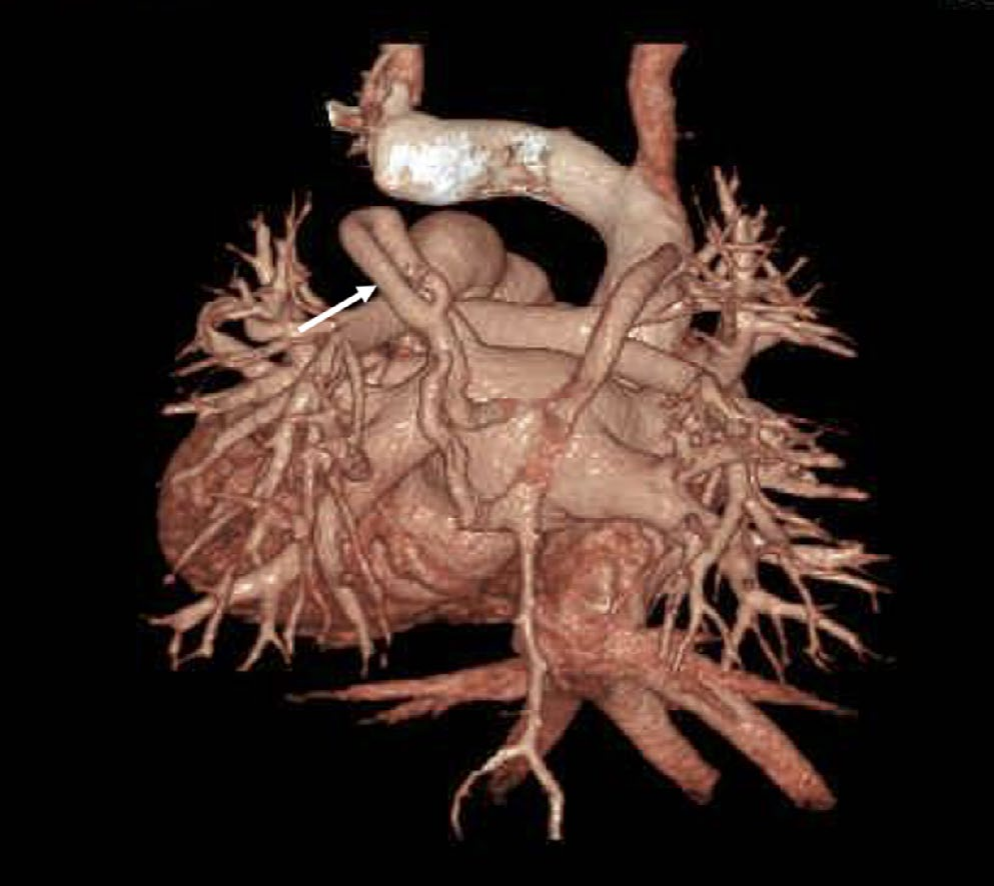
Contrast CT after surgery repair (behind view). White arrow indicates second vertical vein (originated from proximal ligated vertical vein and drain into superior vena cava)
